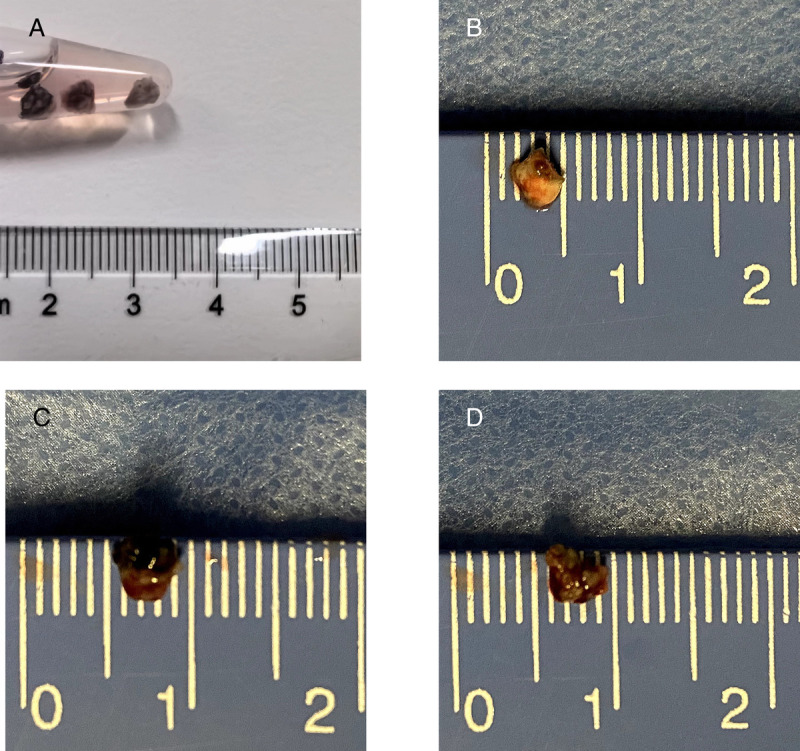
Samples obtained using EBUS-TTCB, with a mean diameter of 5 mm, without any airway tissues attached to them. (A) The overview of the 3 samples obtained by EBUS-TTCB. (B) Sample obtained by the first cryobiopsy (size, 4 mm × 4 mm). (C) Sample obtained by the second cryobiopsy (size, 5 mm × 4 mm). (D) Sample obtained by the third cryobiopsy (size, 5 mm × 4 mm). EBUS-TTCB: Endobronchial ultrasound–guided transbronchial tunnel cryobiopsy.
